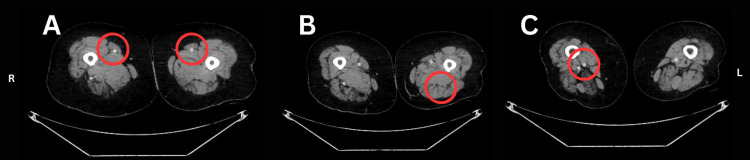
A) Axial CT angiogram at the proximal–mid one-third junction of the thigh, demonstrating superficial femoral arteries (SFA) of normal caliber without luminal narrowing. B) Axial CT angiogram at the adductor canal level, showing a left hypoplastic PSA with significantly reduced luminal diameter relative to the right. C) Axial CT angiogram at the distal one-third of the thigh, just below the adductor canal, where the circled region demonstrates normal caliber of the right SFA and right PSA at the popliteal level.
